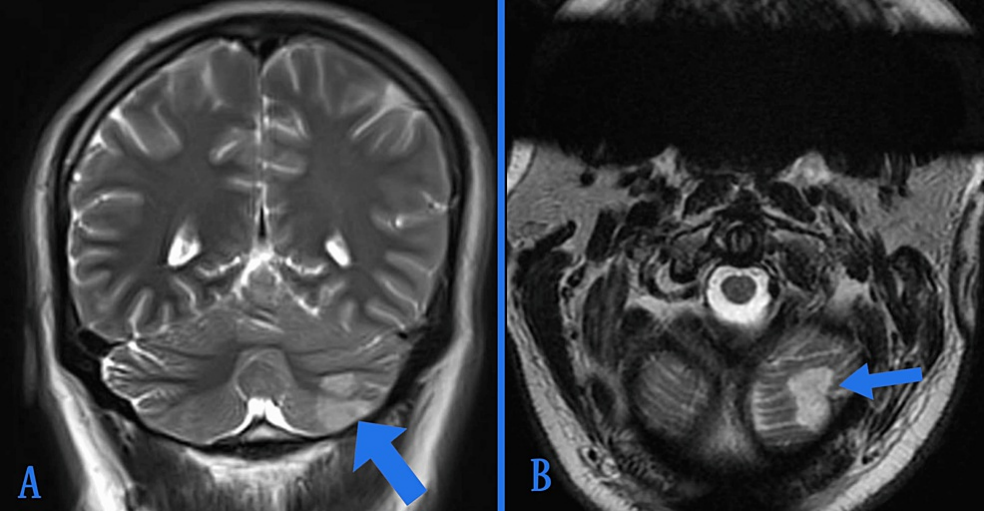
Brain MRI showing a left cerebellar subacute ischemic nonhemorrhagic infarction, pointed with an arrow: (A) coronal cut and (B) axial cut. MRI, magnetic resonance imaging
